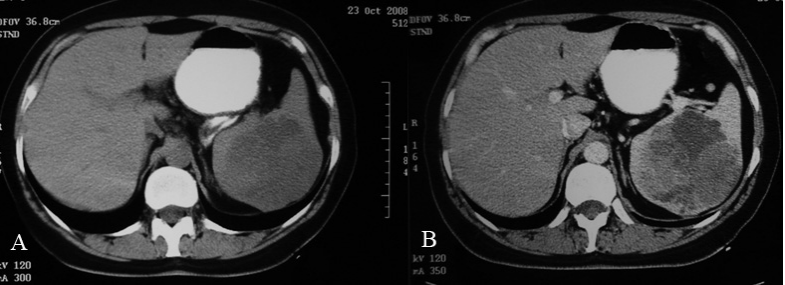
CT unenhanced (a) and contrast enhanced during the early portal venous phase (b) images show an inhomogeneous hypodense well defined mass with some internal enhancement in the spleen.
